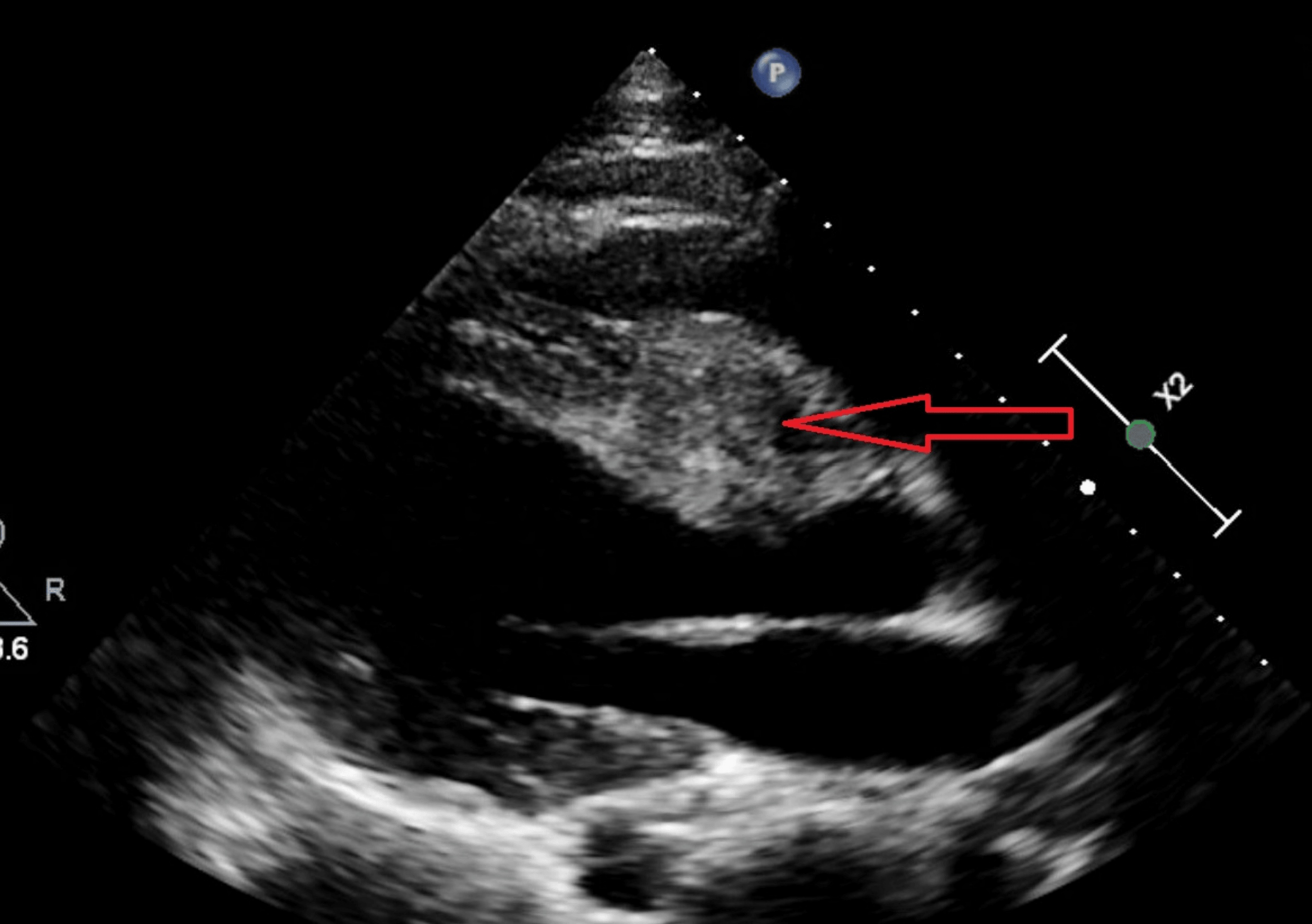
Transthoracic echocardiogram (parasternal long axis view) showing a 5-cm interventricular septal mass
